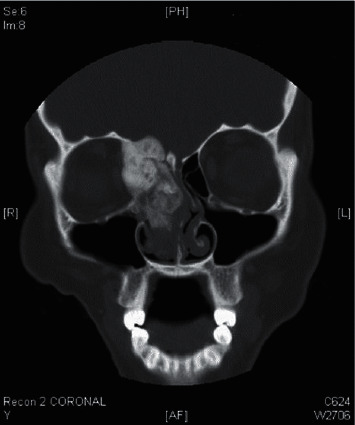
The right ethmoid sinus and nasal space growth encompassing penetration of the orbital as well as cerebral interplanetary are perceived preceding the paranasal coronal CT scan image.
